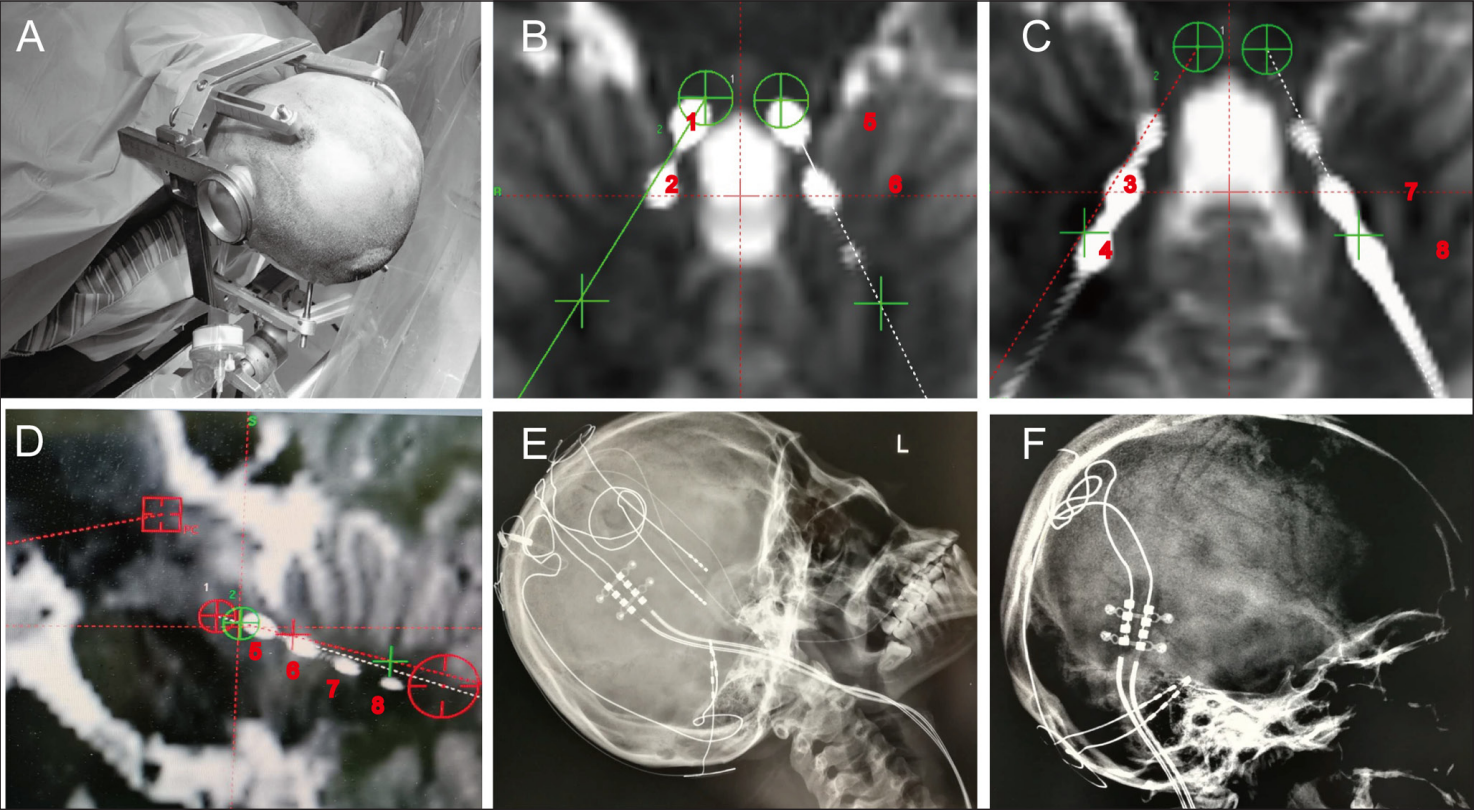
Images showing the surgical procedure as well as the postoperative head images. During the first stage of trial stimulation, a stereotactic frame (Leksell) was installed under local anesthesia, and head computed tomography (CT) scan (Helex Sequence) was performed. Given the location of the target and the suboccipital approach, the head was fixed to the bed with the straight front piece of the Mayfield head rest during prone position for the anterior bar of the frame, and the long posterior fixation posts, which make the stereotactic frame, were placed lower than usual (3A). For the contacts of the leads to traverse both the SCP and DN, the customized DBS lead for research (L303, Beijing Pins Medical Co., Ltd., Beijing, China) was used. The lead featured 4.0 mm spacing between each of the four 3.0 mm electrodes at the distal end; the electrode spread is 24.0 mm with the ventral contact within SCP and the dorsal contact within DN. For the operation, two L303 DBS leads were implanted into the patient in the prone position under general anesthesia according to the planned coordinates and trajectory with the lead connected to the external extension wire for trial stimulation. The bilateral GPi leads were retained during this step of surgery (3E). After 1 week of trial stimulation, we removed the bilateral GPi DBS leads (3F) and connected the SCP DBS leads to the previous implanted pulse generator (G102R, PINS, Beijing Pins Medical Co., Ltd., Beijing, China). A 1.5 T T1- and T2-weighted MRI is allowed for patients with implanted DBS leads according to the manufacturer’s indications. Ventral contacts 1 (or 5 on the left side) and 2 (or 6) were close to the superior cerebellar peduncle (SCP) (3B), and dorsal contacts 3 (or 7) and 4 (or 8) were close to the dentate nuclei (DN) (3C). All four contacts of the left side are shown in (3D). The X-ray image (3E) showed both the new implanted electrodes in the cerebellum and the old electrode in the globus pallidus internus (GPi), while the (3F) showed only the new implanted electrodes in the cerebellum existing after explanted the old electrodes in GPi.
